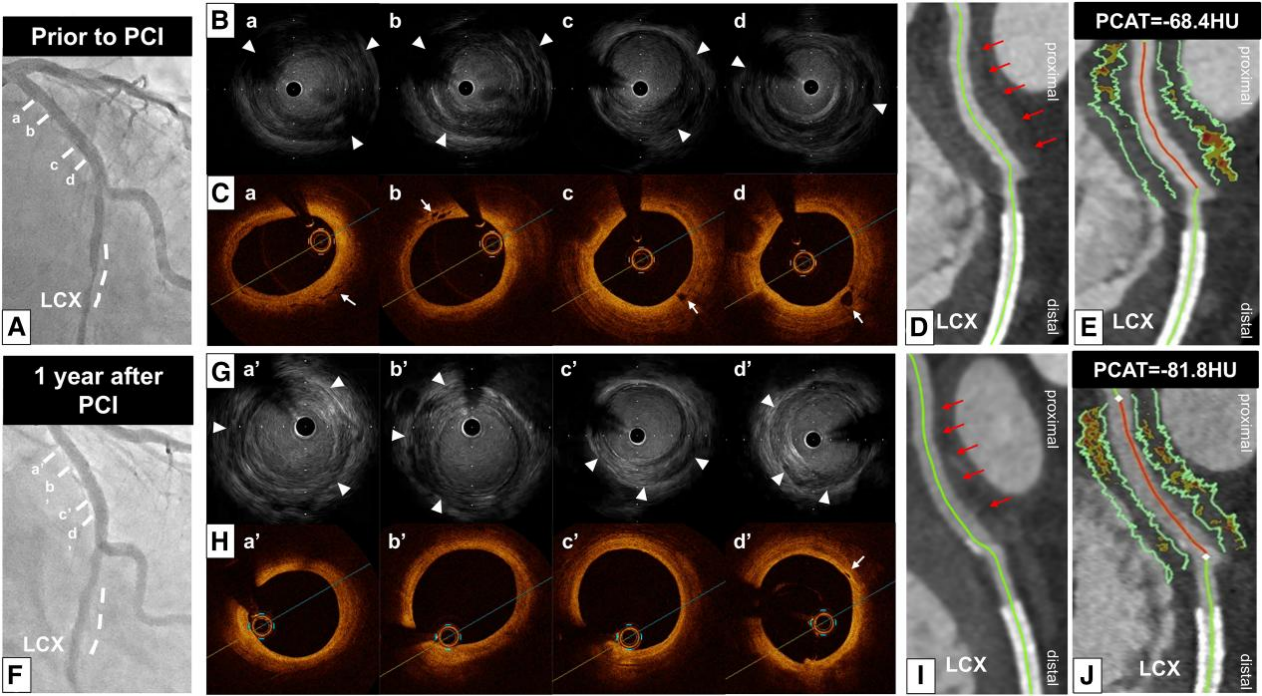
(*A*) Emergent coronary angiography demonstrated severe stenosis in the distal segment of the left circumflex artery (dotted white line). a–d correspond to the intravascular ultrasound and optical coherence tomography images in *B* and *C*. (*B*) Intravascular ultrasound imaging revealed thickening of the adventitia (white arrowheads) at the proximal segment of the left circumflex artery. (*C*) Optical coherence tomography imaging showed vasa vasorum at the proximal segment of the left circumflex artery (white arrows). (*D*) Coronary computed tomography angiography imaging 12 days after primary percutaneous coronary intervention demonstrated soft tissue proliferation surrounding the proximal segment of the left circumflex artery. (*E*) The average pericoronary adipose tissue attenuation at this segment was −68.4 HU. (*F*) Coronary angiography at 1 year following the commencement of prednisolone did not identify any progression of coronary artery stenosis. The dotted white line indicates the segment where a stent had previously been implanted. (*G*) A reduction in the thickness of the adventitia (white arrowheads) was observed on intravascular ultrasound imaging. (*H*) A reduction in the vasa vasorum was observed on optical coherence tomography imaging (white arrow). (*I*) Coronary computed tomography angiography imaging demonstrated a reduction in soft tissue volume. (*J*) Pericoronary adipose tissue attenuation decreased to −81.8 HU. IVUS, intravascular ultrasound; HU, Hounsfield units; LCX, left circumflex artery; OCT, optical coherence tomography; PCAT, pericoronary adipose tissue; PCI, percutaneous coronary intervention.
